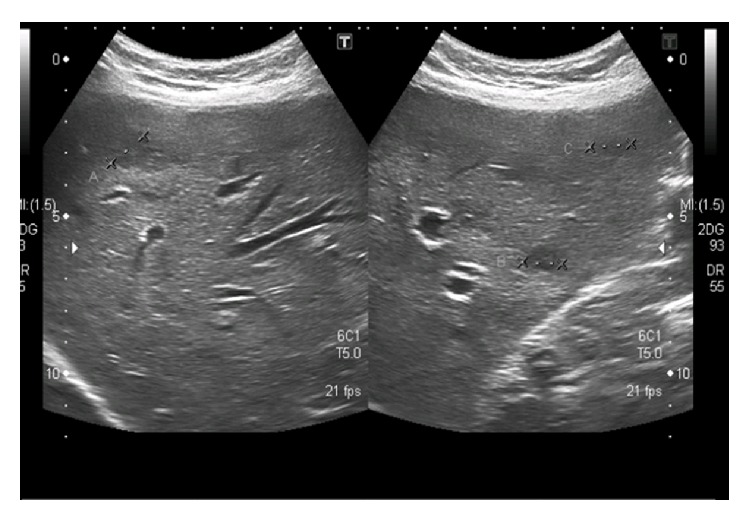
Ultrasound upper abdomen. Multiple hypoechoic tiny lesions on both lobes, few of them cystic in appearance.
